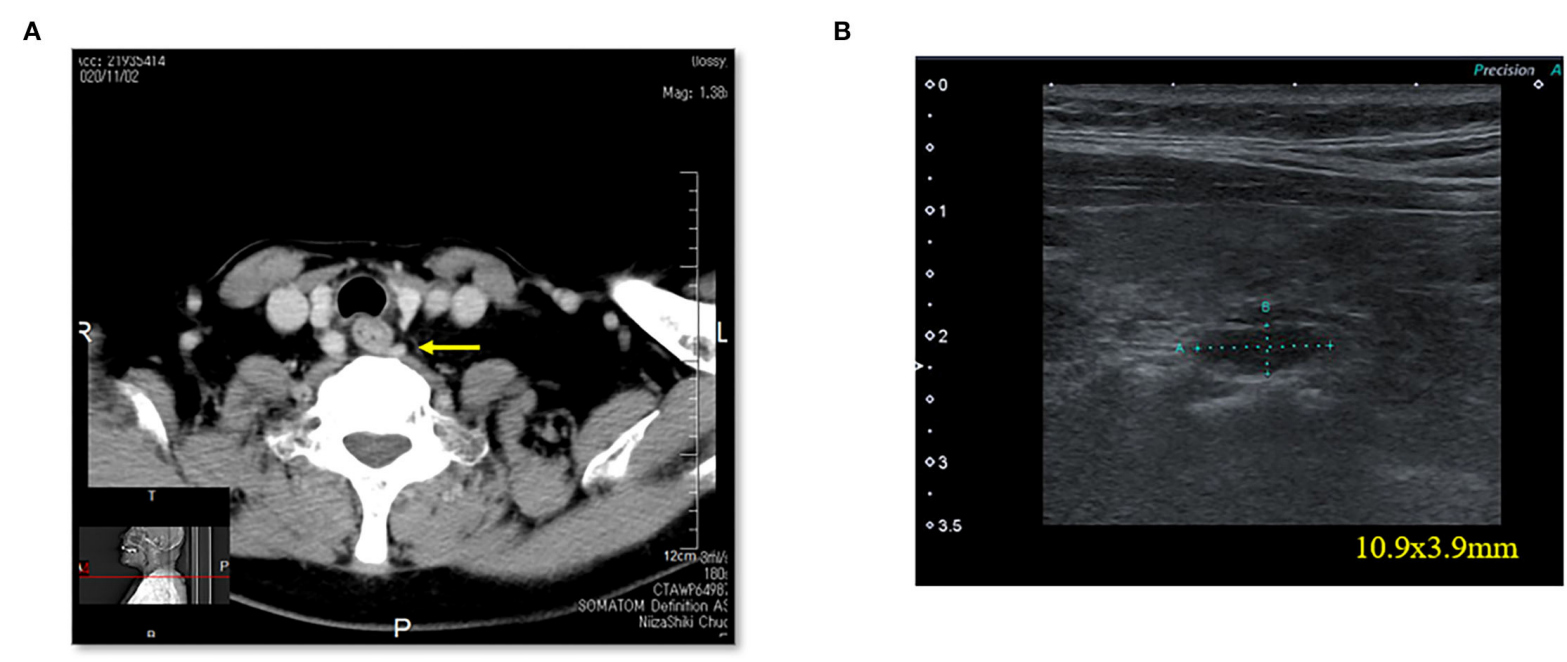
Case 6. (A) Thoracic enhanced CT scan showing a mass (EPA: yellow arrow) near the cervical esophagus. (B) Cervical neck ultrasound showing a mass (10.9 × 3.9 mm [EPA]) near the cervical esophagus.
